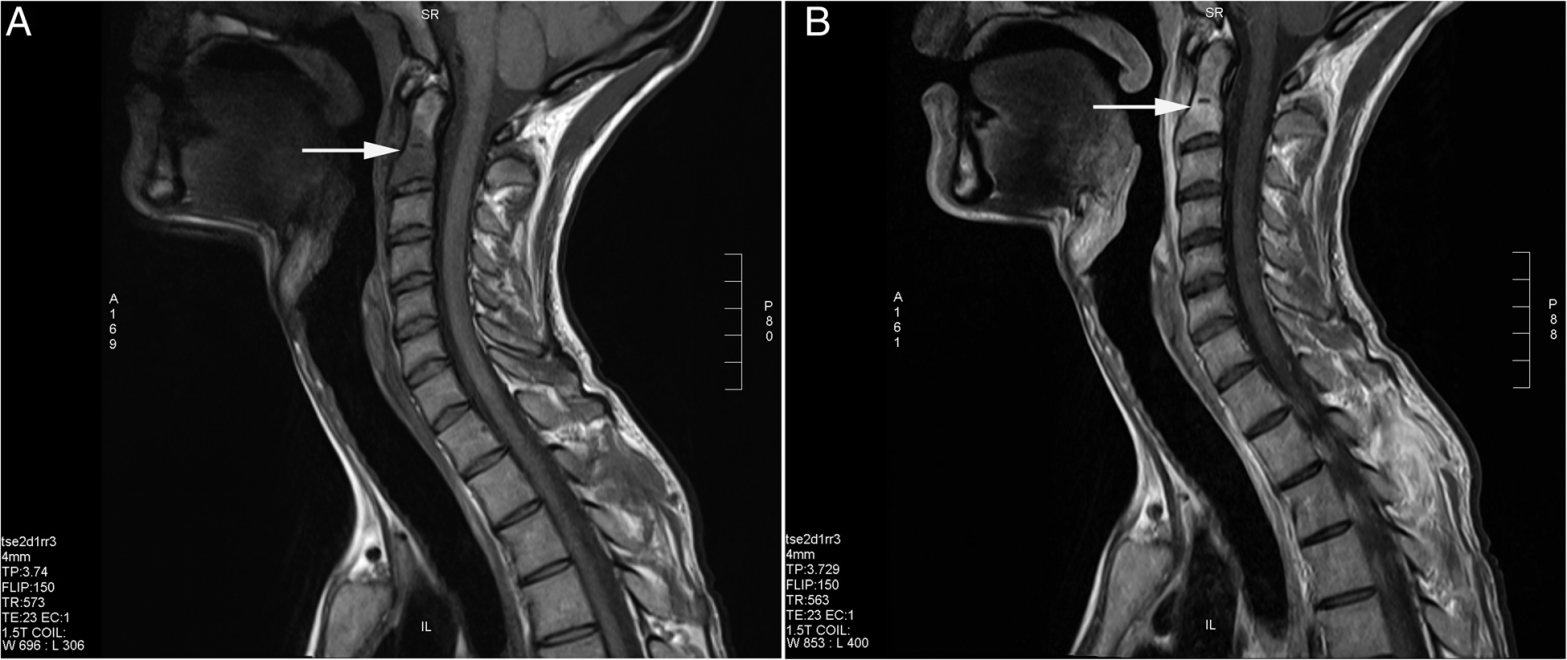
MRI images (T1 sequences) of the affected cervical vertebra (white arrows) before (A) and after (B) contrast enhancement.
